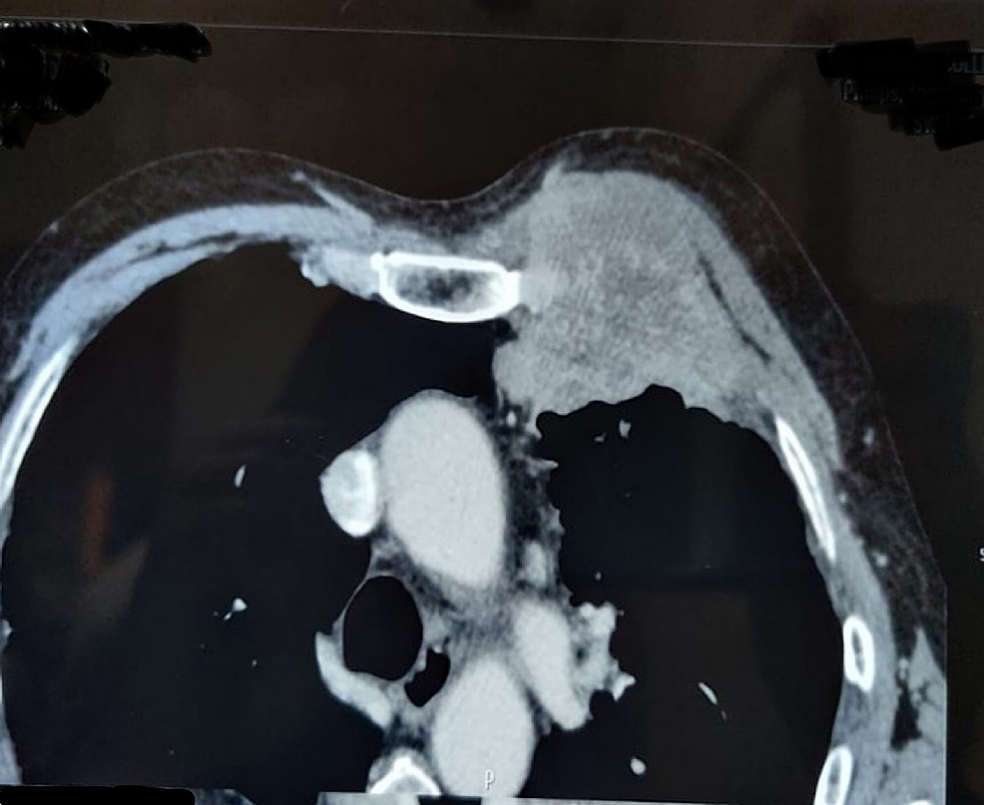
CECT chest showing chest wall infiltration by an ill-defined mass arising from left lung parenchyma. CECT: contrast-enhanced CT.
